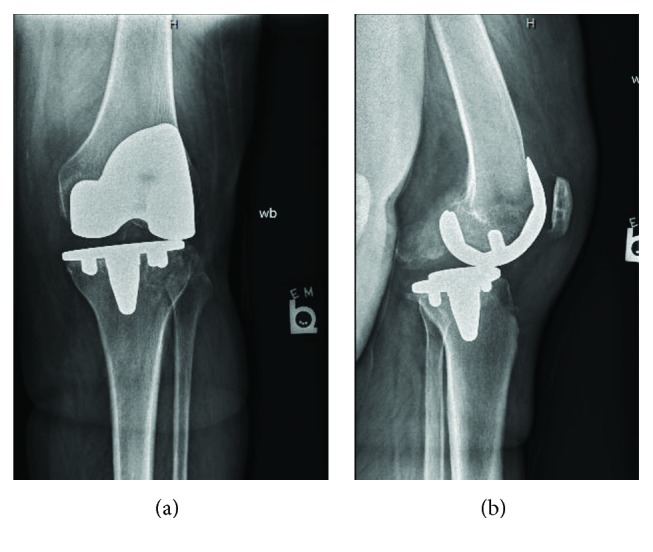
(a, b) AP and lateral radiographs of the knee indicating posterior dislocation of the tibia and instability of knee prosthesis. Note inability to ascertain the direction of the rotating platform.
